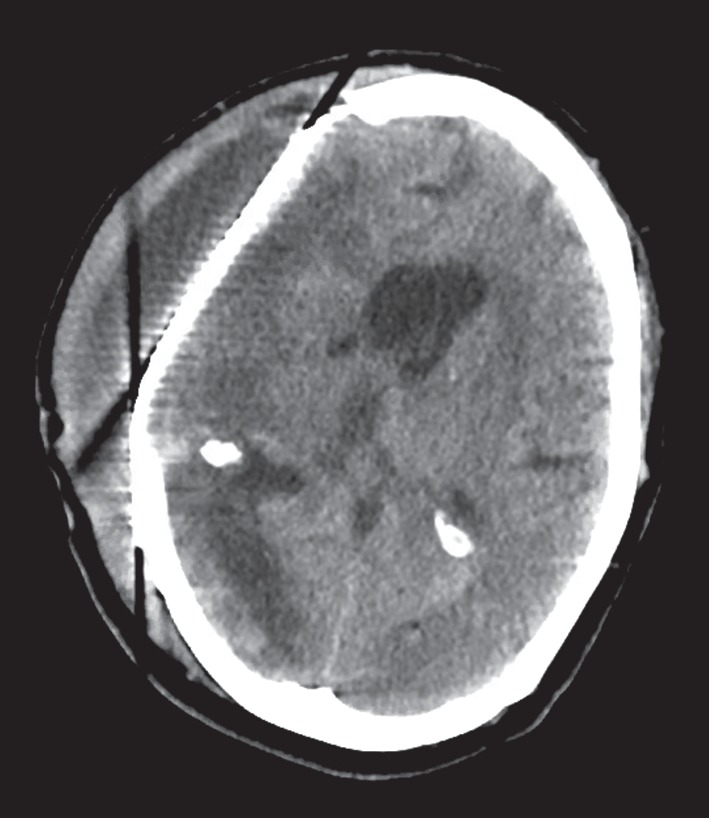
Repeat head CT was performed identifying an enlarging subgaleal fluid collection measuring 14 × 23 mm.
